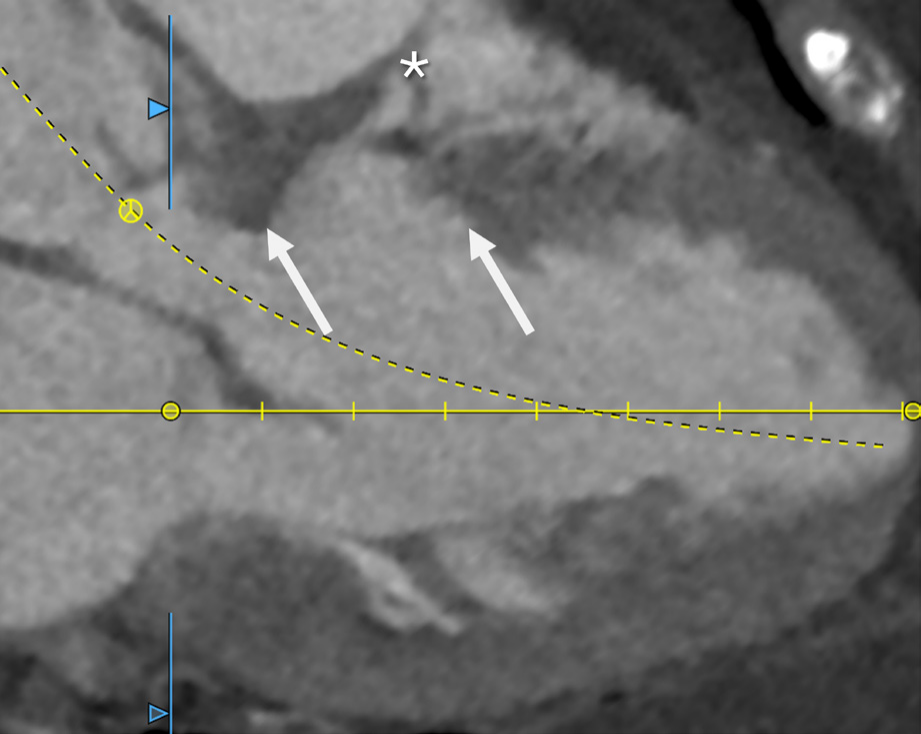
Computed tomography after Septal Scoring Along Midline Endocardium shows the lengthwise extent (white arrows) of the myotomy, along with a ventricular septal defect (white asterisk). The complex ventricular septal defect pattern is more consistent with steam pop than with excessively deep myotomy.
